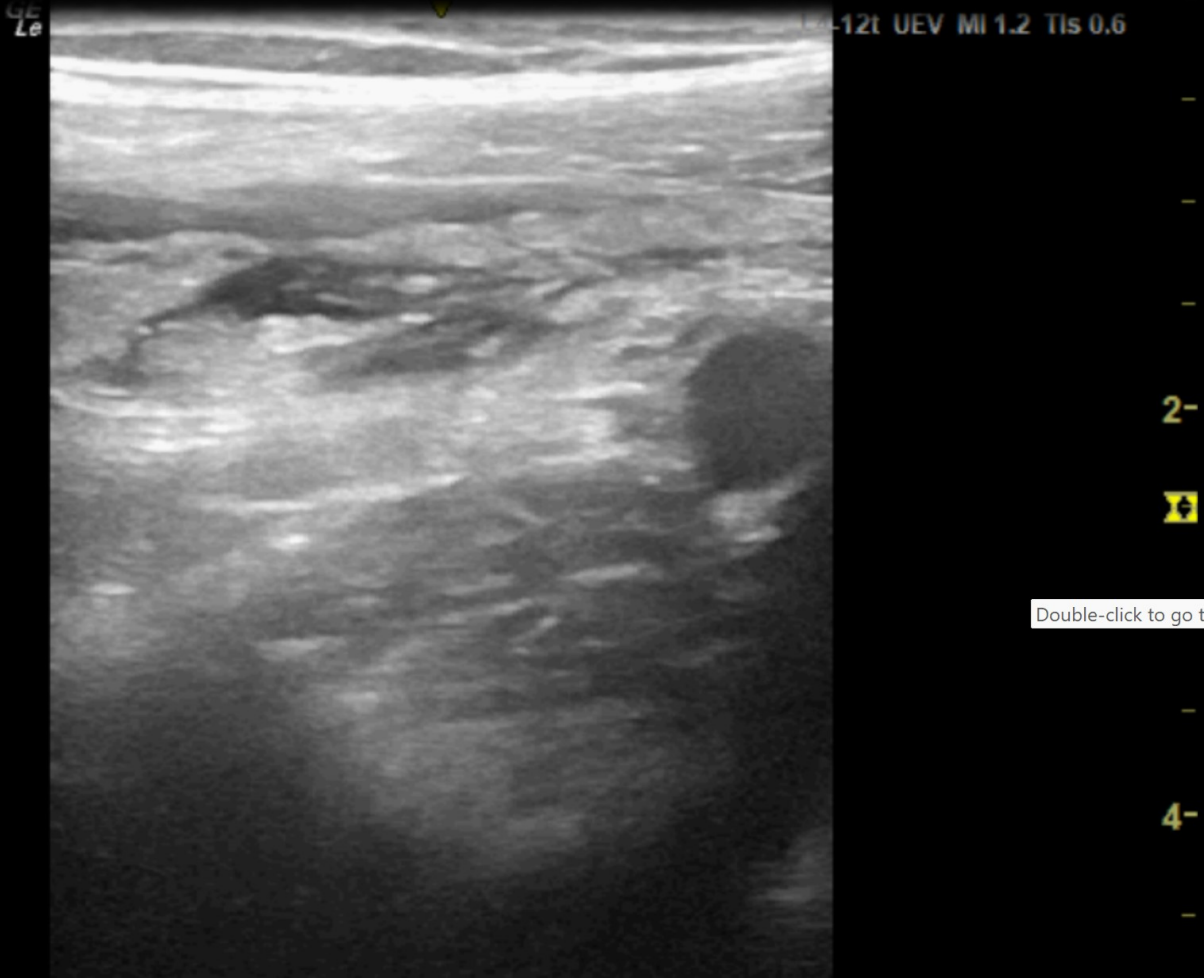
Point of care ultrasound (POCUS) image of right iliac fossa using the high frequency 12 MHz linear probe. This shows a moderate degree of echogenic inflammatory changes felt to be consistent with fat wrapping. There is small region of localised free fluid within this.
